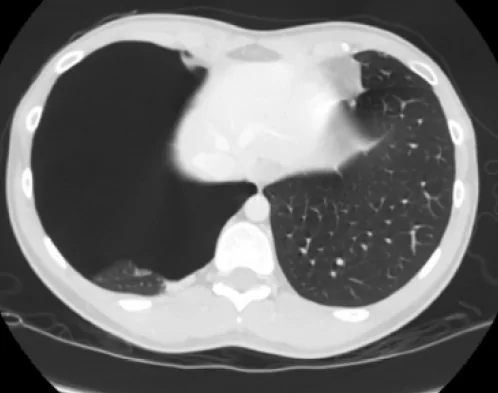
Computed tomography demonstrating incidental right-sided pneumothorax in a thin young man.
